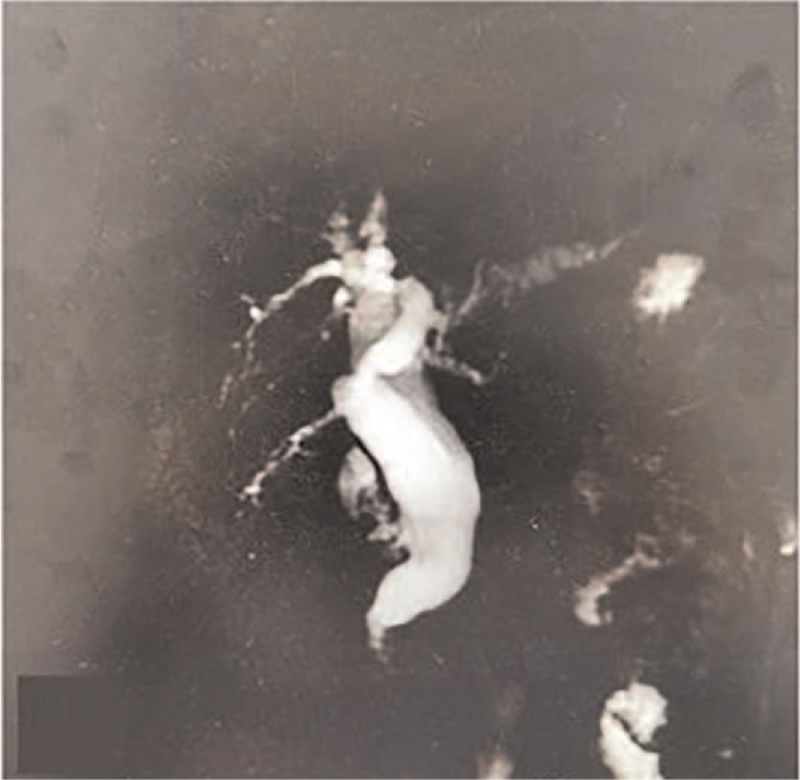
MRI showed extrahepatic ductal dilatation in the common bile duct region.
